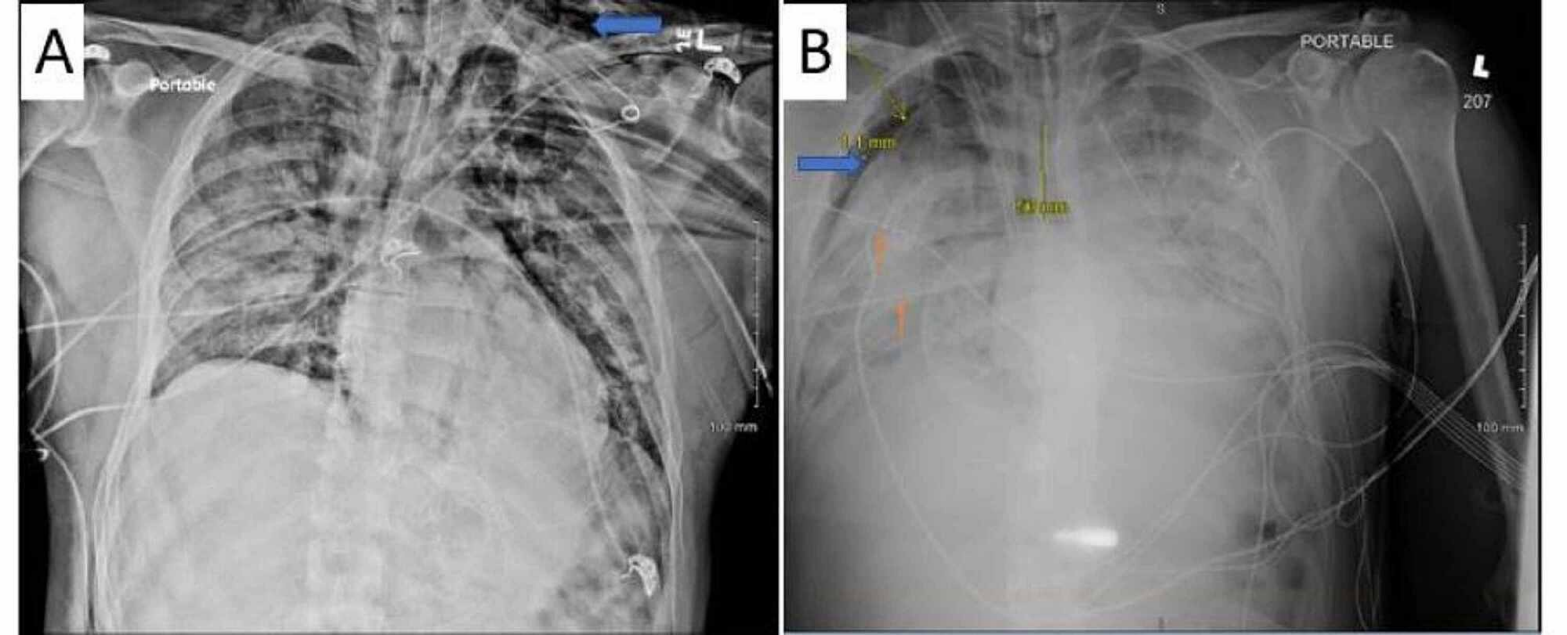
(A) Chest radiograph showing left sided subcutaneous emphysema {blue arrow} (B) Persistent right sided pneumothorax (blue arrow) even with placement of two right sided chest tubes (orange arrows). Also noted on this image is the right internal jugular extracorporeal membrane oxygenation (ECMO) cannula.
